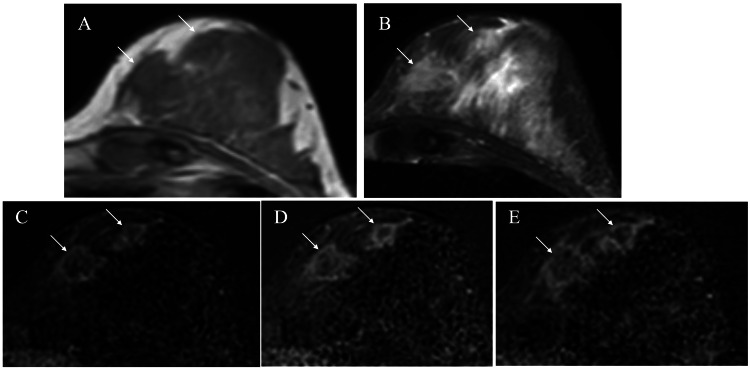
Magnetic resonance imaging (MRI) findings MRI of the masses (arrows) showed low signals on T1-weighted images (A), slight high signals on fat-suppressed T2-weighted images (B), and retained rim enhancement (C: early, D: mid, and E: late phases) on subtraction images.
